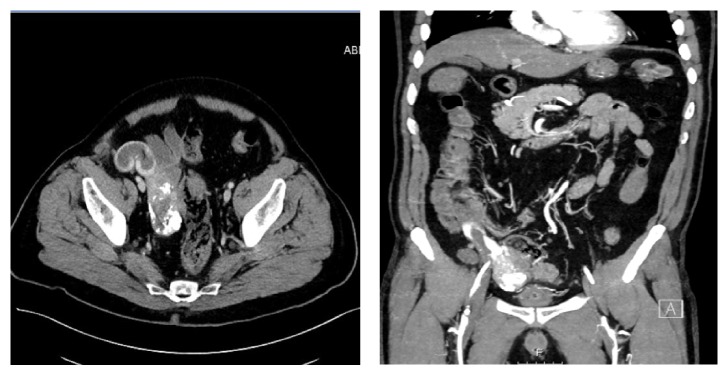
Axial and coronal CT angiography of the abdomen showing a calcified mass at the right pelvis, attached to the distal ileum with intraluminal contrast blush.
